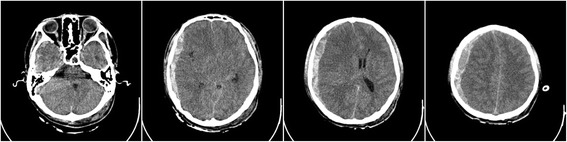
Admission computed tomography showing right acute subdural hematoma with midline shift
